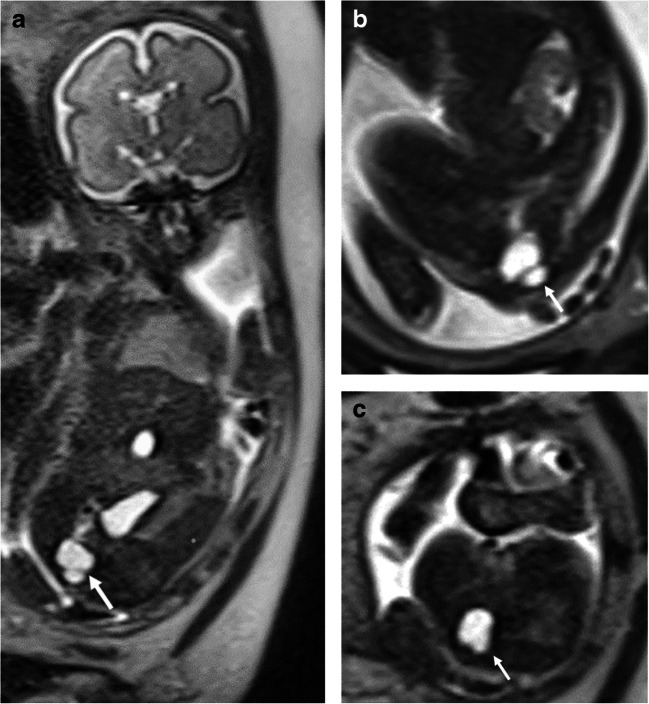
A male fetus at 26 weeks’ gestation with presacral lymphatic malformation (N20, Table 3). a–c Coronal oblique (a), sagittal paramedian (b) and axial (c) T2-weighted MR images (repetition time/echo time 1,200/90 ms) show the presacral fluid isointense lesion (*arrows*) with an internal septum. The prenatally suggested diagnosis was a presacral teratoma, based not only on imaging findings but also the typical anatomical location of this common congenital tumor. Pathology after postnatal surgery identified a macrocystic lymphatic malformation
